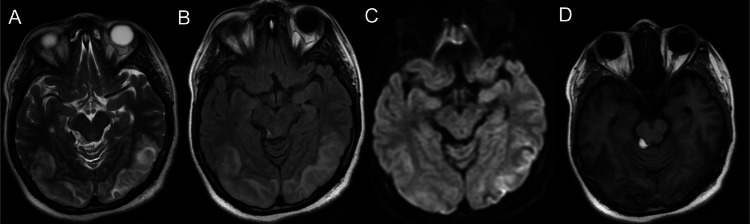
MRI at the level of the midbrain. The altered signal intensity which appears hyperintense on T2WI (A), FLAIR (B); T2 shine through in DWI (C); hypointense on T1WI (D) involving bilateral posterior temporal and temporo-occipital regions. An incidental midbrain lipoma of size 11.6 X 8mm is noted which is hyperintense on T1WI (D). DWI - Diffusion-weighted imaging FLAIR - Fluid attenuated inversion recovery MRI - Magnetic resonance imaging T1WI - T1 weighted imaging T2WI - T2 weighted imaging
